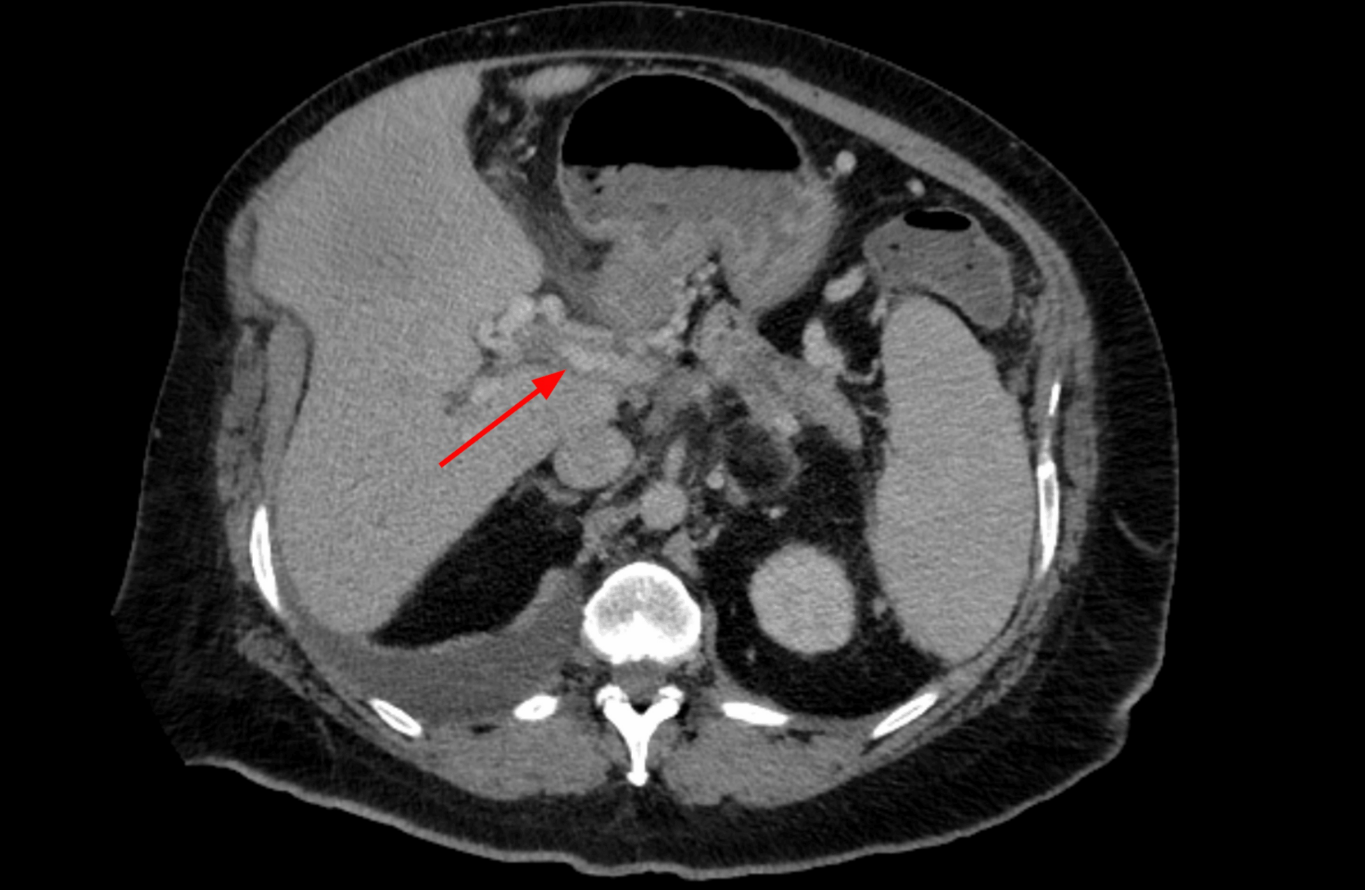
CT abdomen pelvis showing chronic inflammatory changes of the pancreas. Chronic inflammatory changes of the pancreas. CT: computed tomography
